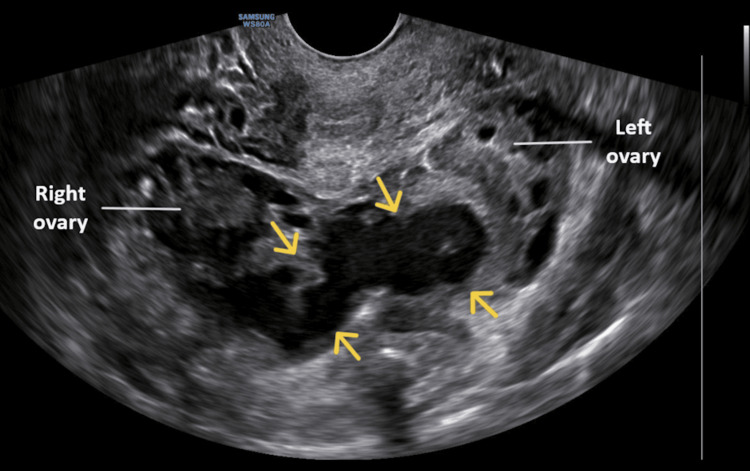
Transvaginal ultrasound imaging of the periappendiceal abscess misdiagnosed as pyosalpinx The presence of a tubular structure in the true pelvis (indicated by yellow arrows) led to the misdiagnosis of a pyosalpinx.
